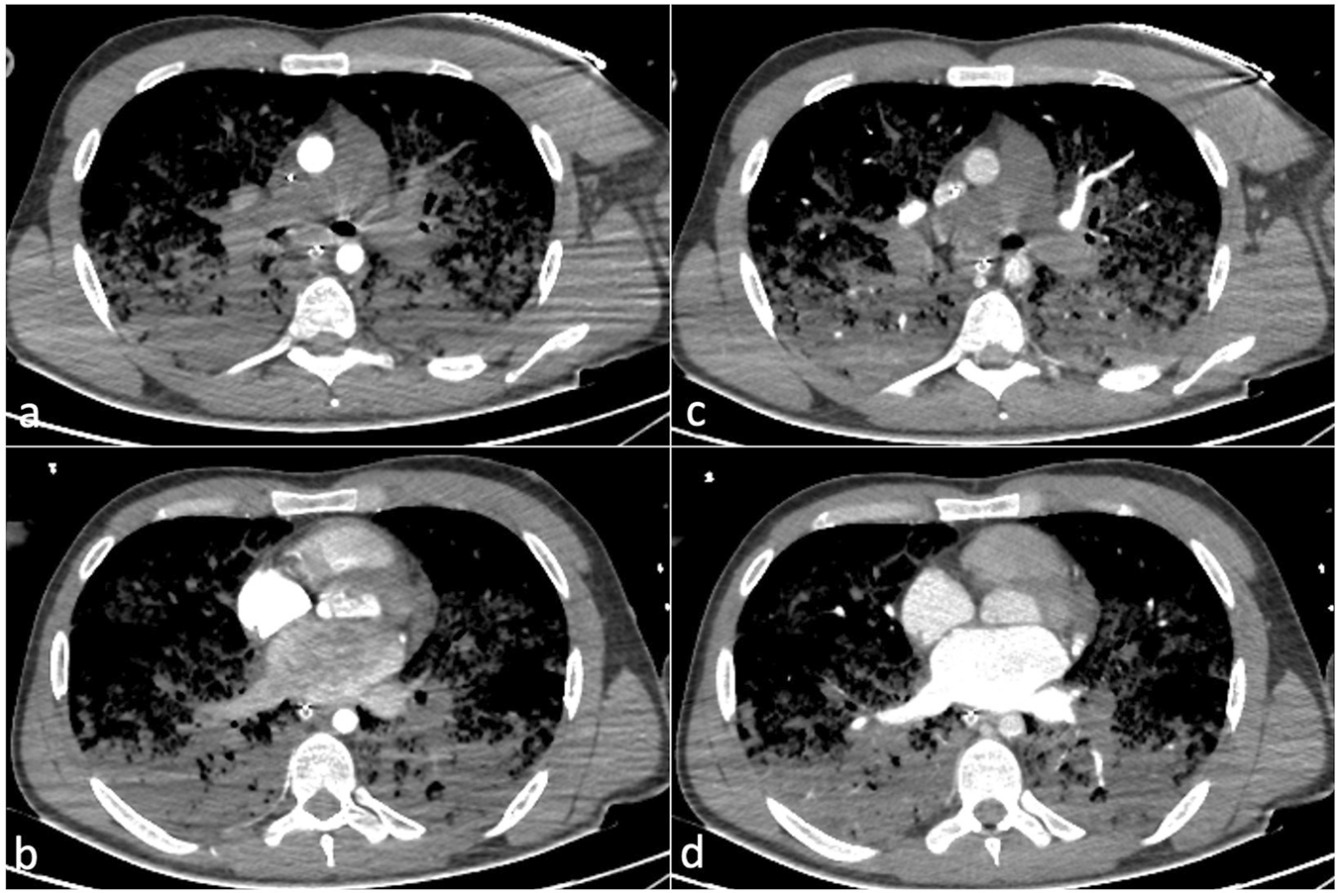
Axial contrast-enhanced computed tomography (CT) images in the arterial (a, b) and venous phases (c, d) in an acute respiratory distress syndrome (ARDS) patient on veno-arterial (VA) extracorporeal membrane oxygenation (ECMO) therapy with severe heart failure. At the main pulmonary artery bifurcation (a, c), there is no enhancement in the pulmonary arteries, while at the left atrium level (b, d), retrograde filling of the left atrium and pulmonary veins is seen due to the VA-ECMO system
